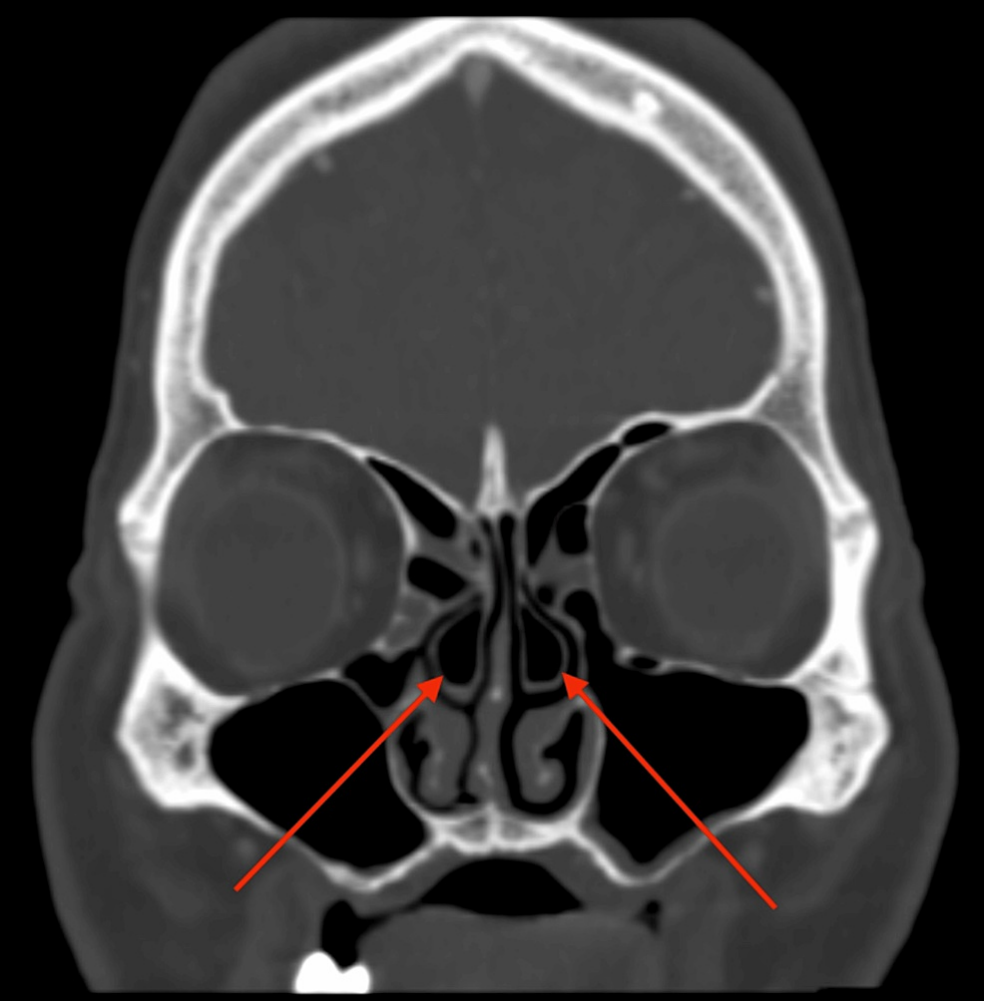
CT scan of the paranasal sinuses in coronal plane in bone window The image shows bilateral concha bullosa (red arrows).
